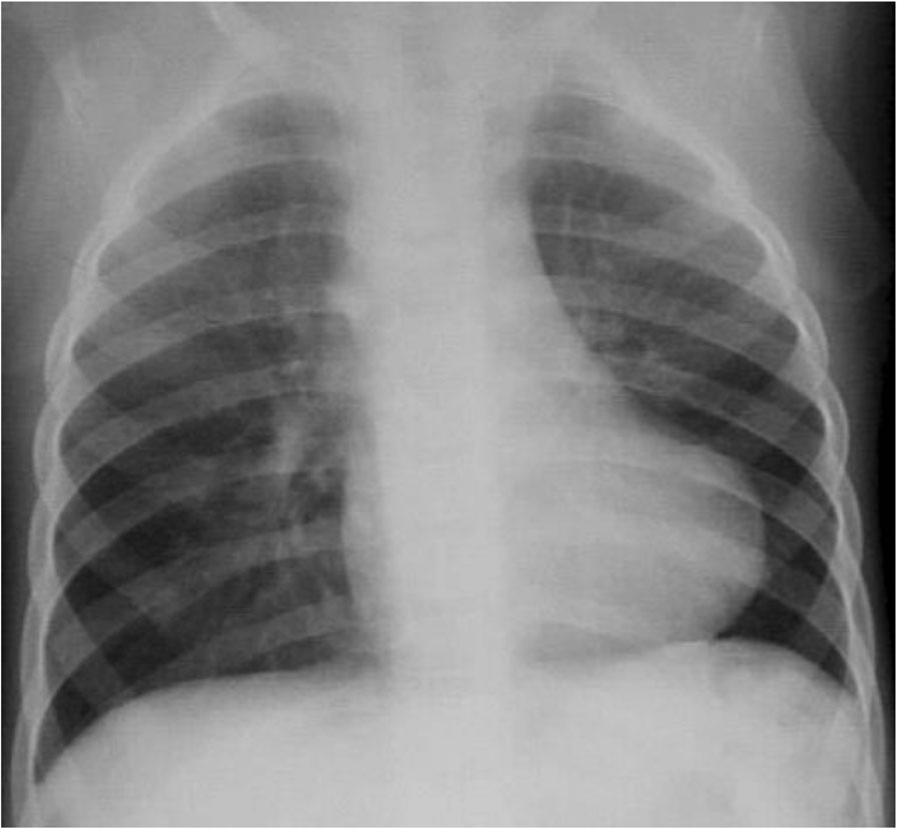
Chest radiograph of child with TOF showing the classical “boot-shaped heart” due to the narrow mediastinum and RV hypertrophy with decreased pulmonary vascular markings
